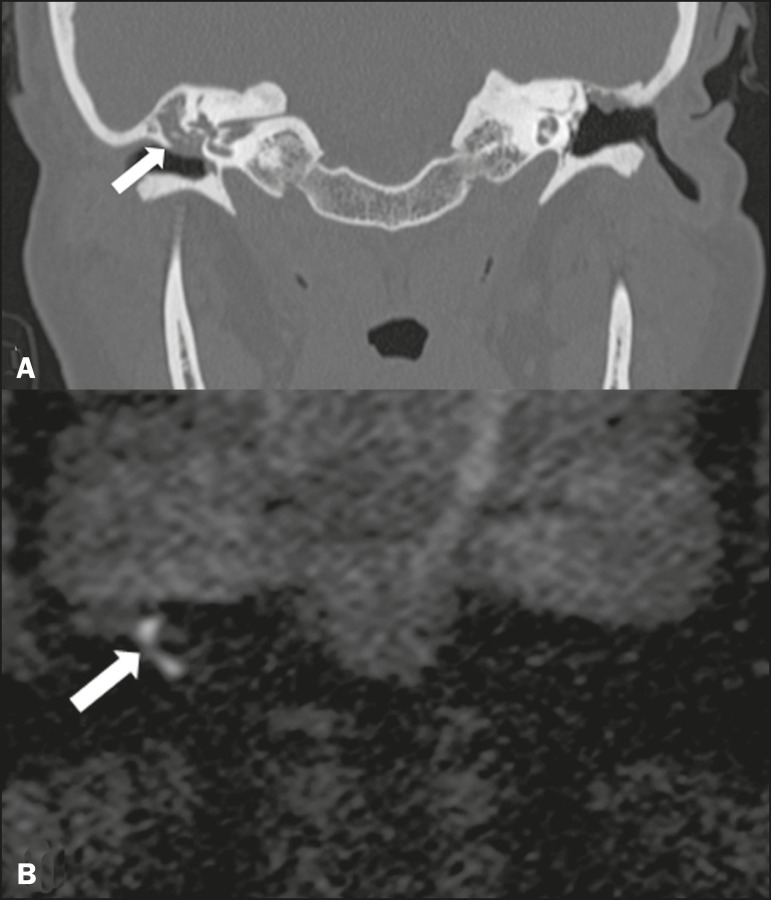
Cholesteatoma. A: Coronal CT scan, with bone window
